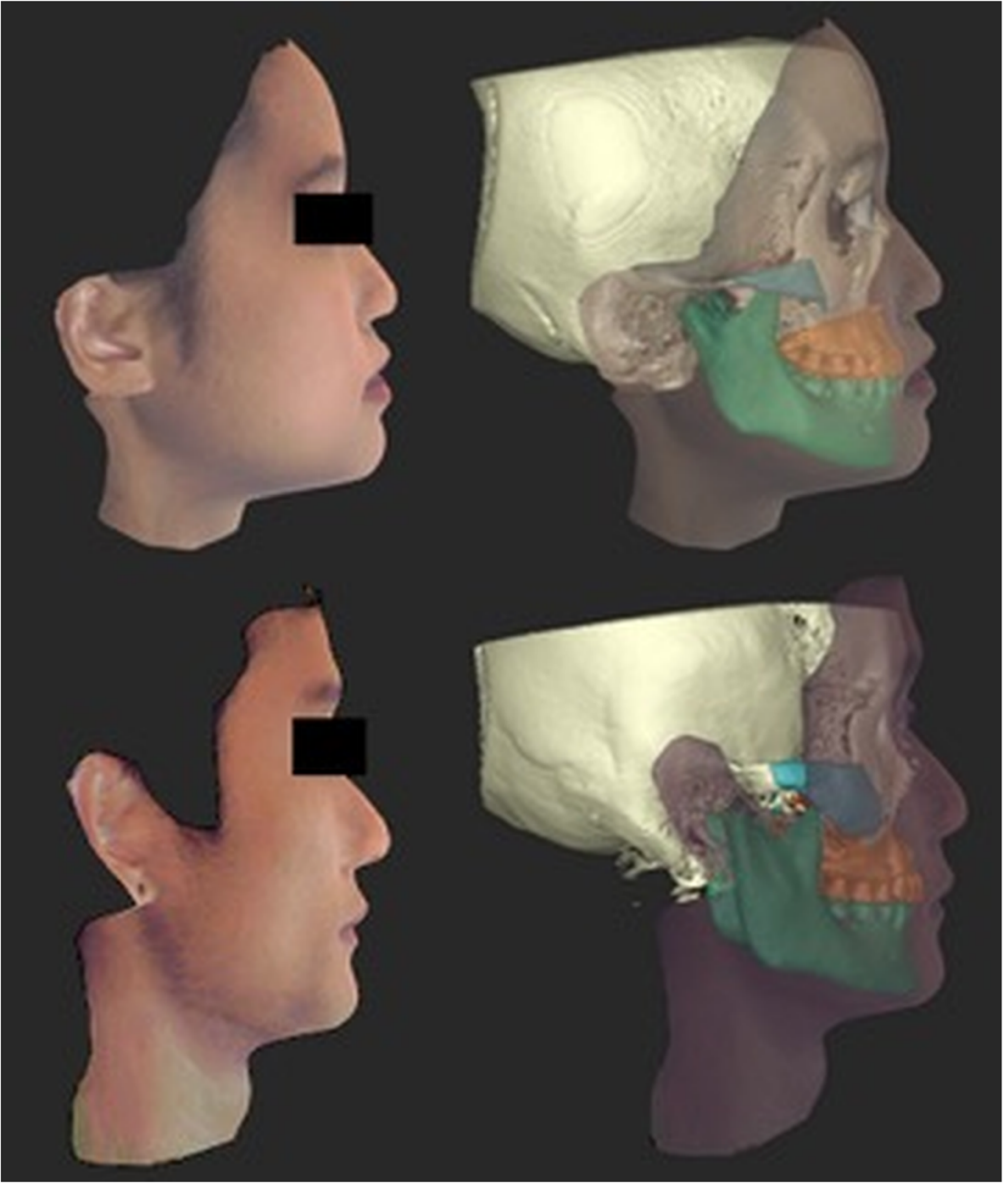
Superimposition of facial 3D images on CBCT images
